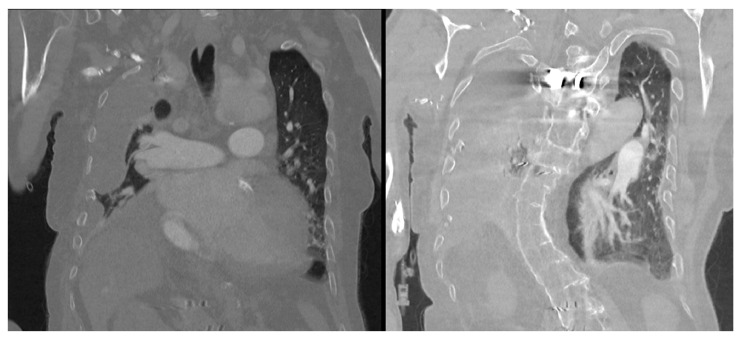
Preoperative chest CT scan showing scoliosis, metallic artefacts from prior spinal surgery, and dilatation of the right heart chambers.
